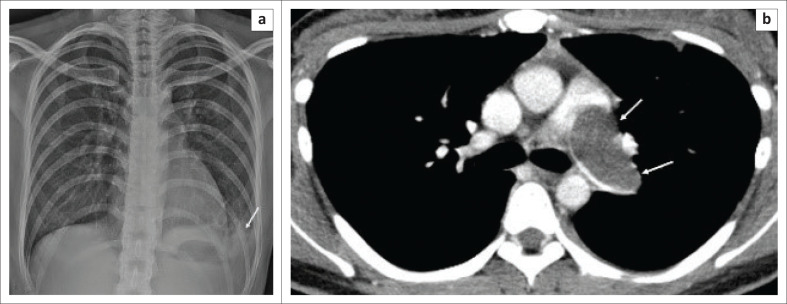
(a) Frontal chest radiograph demonstrates normal sized main, right and left pulmonary arteries with a small left basal pleural effusion (arrow). (b) Axial computed tomography pulmonary angiography demonstrated a filling defect extending from a dilated main pulmonary artery into the left pulmonary artery (arrows).
